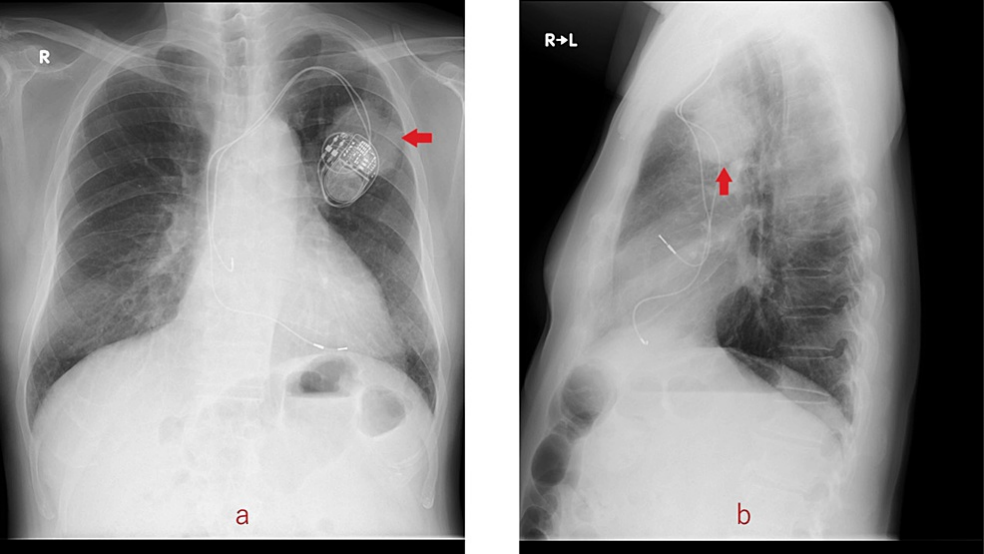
Chest X-ray film. Arrows show a tumor in the left upper lobe of the lung just behind the implanted pacemaker (a: frontal; b: lateral (right to left)).
